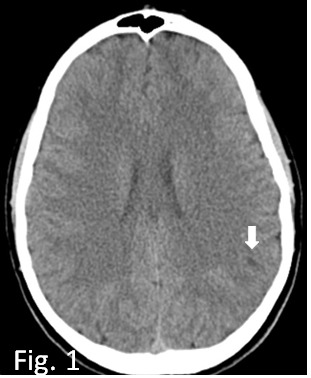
Axial CT basal scan at the onset; slight millimetric hypodensity
